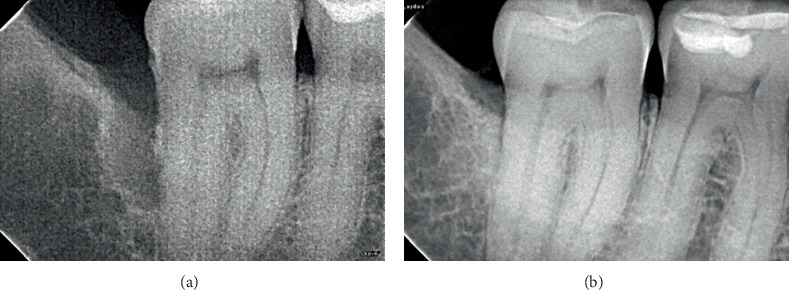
Clinical presentation of Case 5. (a) Periapical radiograph showing severe vertical bone loss extending to two-thirds of Tooth #47. (b) Six-year postoperative radiograph demonstrating improved bone support in the distal area.
